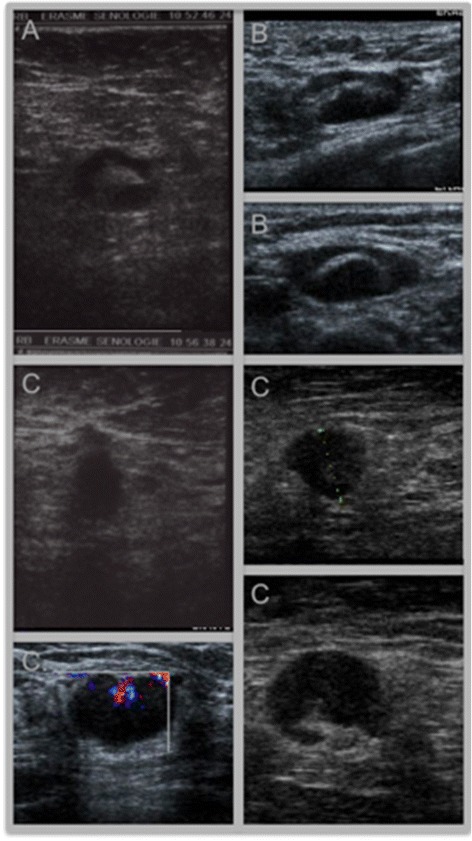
Sonographic images of a minimally suspect axillary lymph node “+” (A), a mildly suspect axillary lymph node “++” (B) and highly suspect axillary lymph node “+++” (C)
